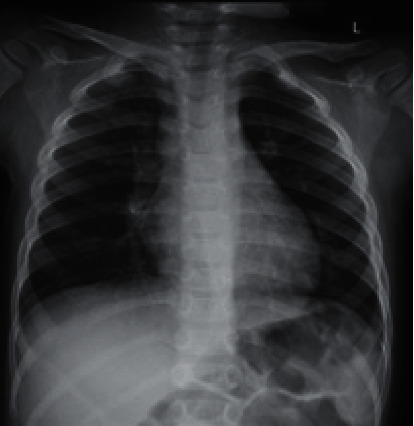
Follow-up chest radiograph obtained 6 months after hospitalization. Frontal CXR has no apparent abnormality.
